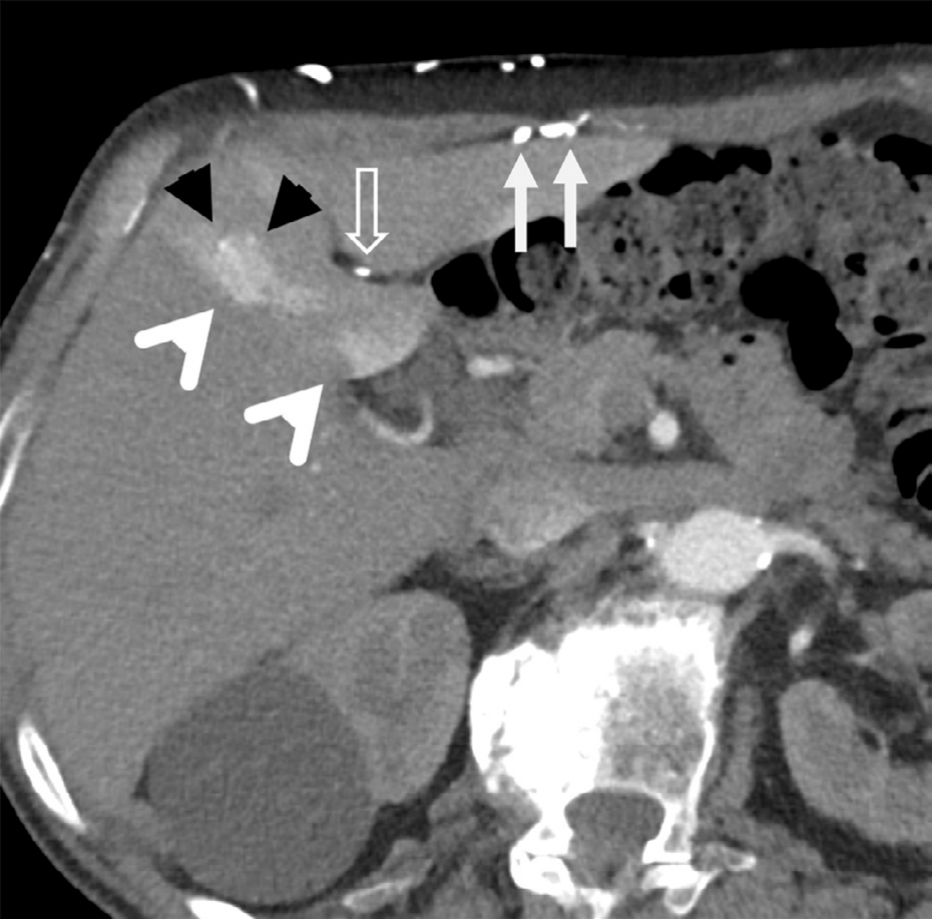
Axial liver CT highlighting segment 4B and collateral vessels.
